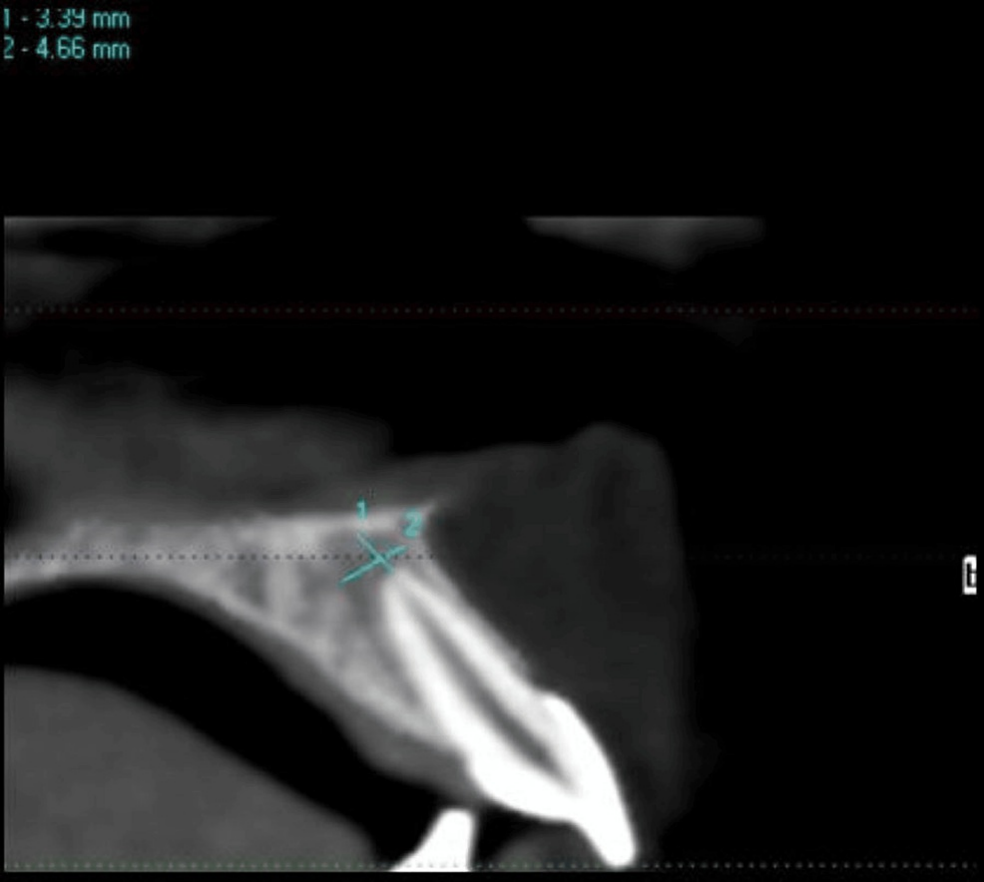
Sagittal CBCT image Sagittal CBCT section showing a hypodense lesion at the periapex of #22. CBCT: Cone beam computed tomography.
